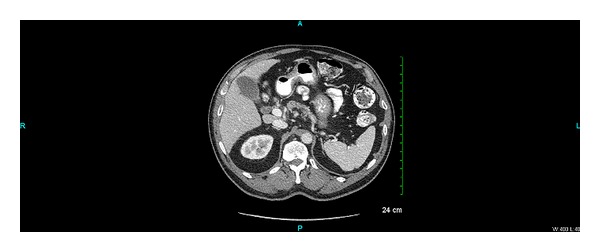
Abdominal and pelvic CT scan showing normal sized liver and spleen.
